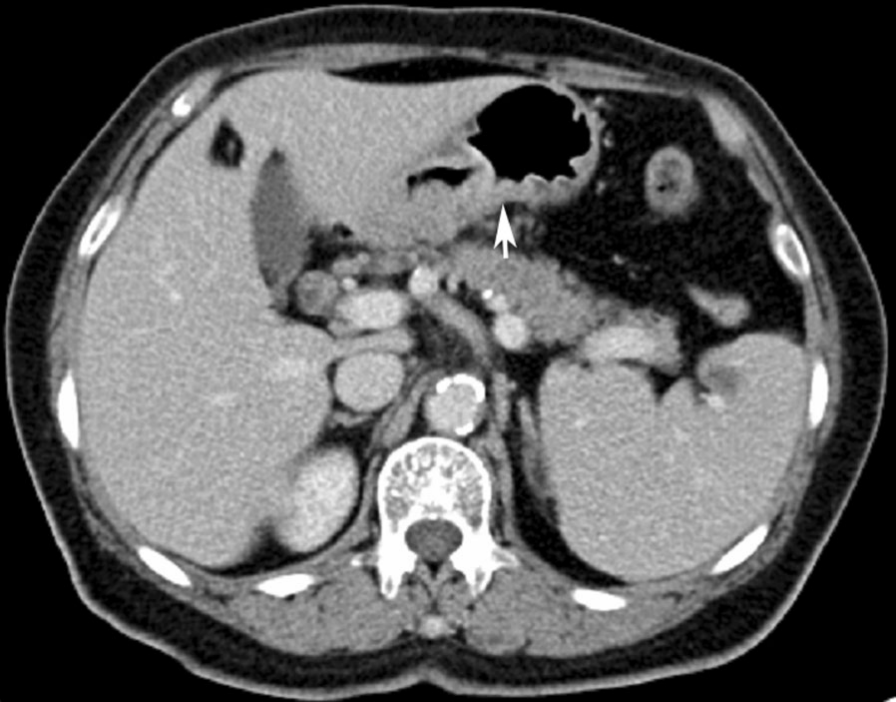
Computed tomography (CT) imaging of the abdomen before surgery showing a local thickened gastric wall in a 77-year-old woman. Thickening of the gastric antrum (*white arrow*)
